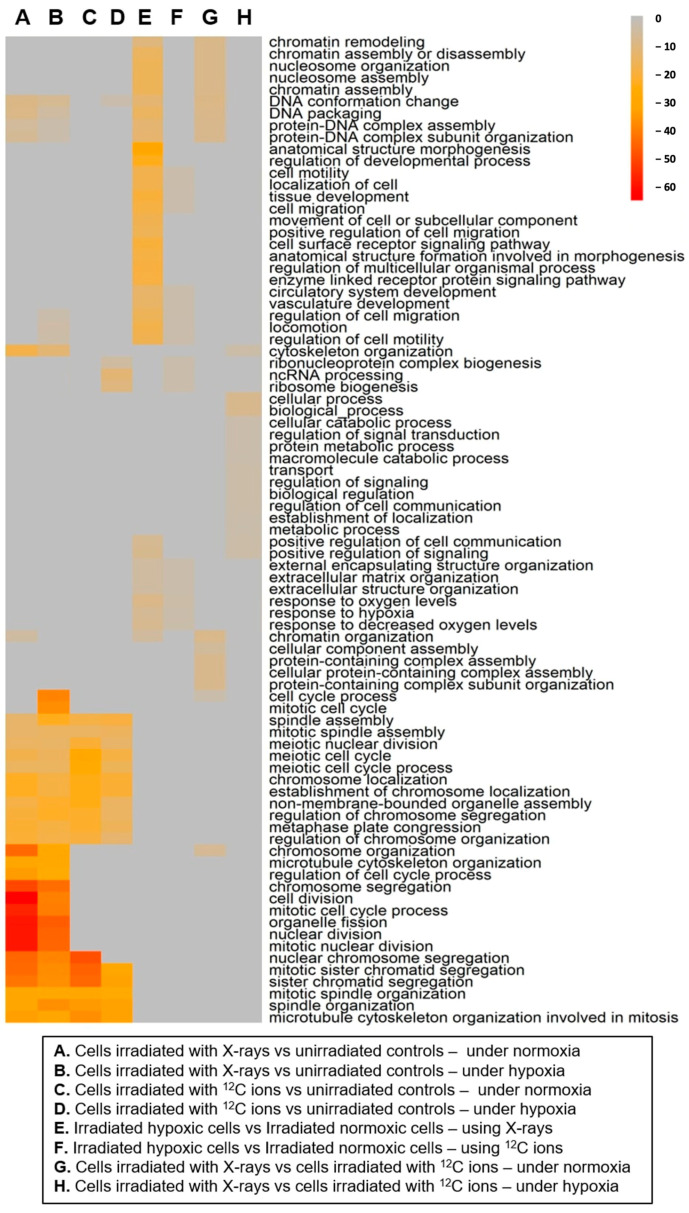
GO terms of differentially expressed genes in A549 cells 4 h after X-rays and ^12^C ion exposure (8 Gy) under normoxia and hypoxia, determined by RNA sequencing. Gene expression was compared for irradiated cells vs. unirradiated controls under normoxia (A and C) and hypoxia following X-rays (A and B, respectively) and ^12^C ions (C and D, respectively) exposure. Furthermore, the effects of oxygenation status on the response to X-rays (E) and ^12^C ions (F) exposure were determined by comparing respective irradiated samples. The radiation quality’s effect was evaluated by comparing the response to X-ray vs. ^12^C ion exposure under normoxia (G) and hypoxia (H). The top 15 GO terms were selected by *p*-value for each comparison. Color intensity represents the logarithm of the adjusted *p*-values, while gray means that the pathway was not significantly enriched in GSEA for this comparison.
